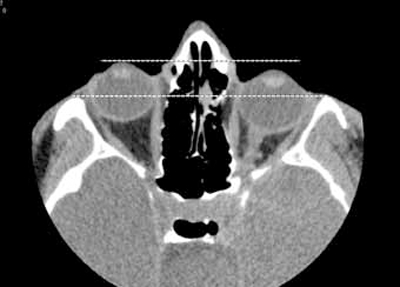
In contrast to the clinical picture, this Axial CT scan image, performed during an inadvertent Valsalva manoeuvre, shows only minimal left enophthalmos (highlighted by the broken lines).
